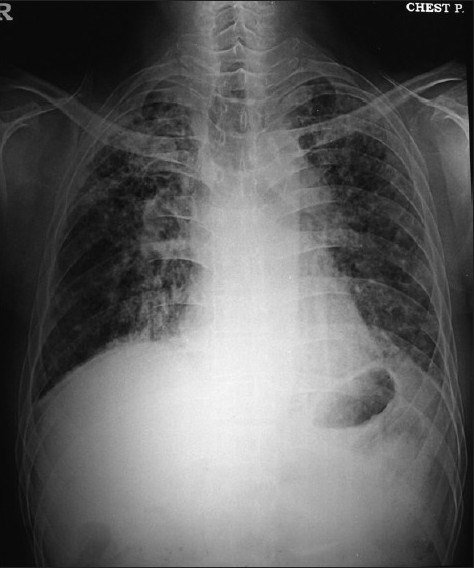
Chest X-ray (2007) shows exudative interstitial nodular and fibrotic pattern
